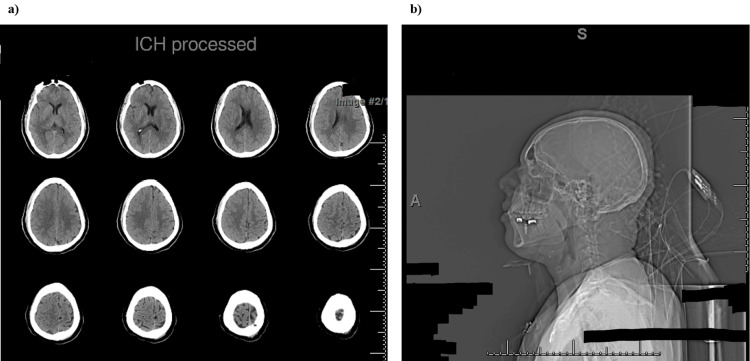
CT of the head and cervical spine. (a) Non-enhanced axial CT imaging through the head shows no evidence of intracranial hemorrhage (ICH) or cerebral edema. Gray-white matter differentiation is preserved. The ventricles are normal in size. The basal cisterns are patent. The calvarium is intact. (b) Non-enhanced CT imaging through the cervical spine shows maintained cervical vertebral body heights. There is no evidence of acute fracture. There is also no prevertebral edema or paraspinal hematoma. Spinal alignment is normal.
